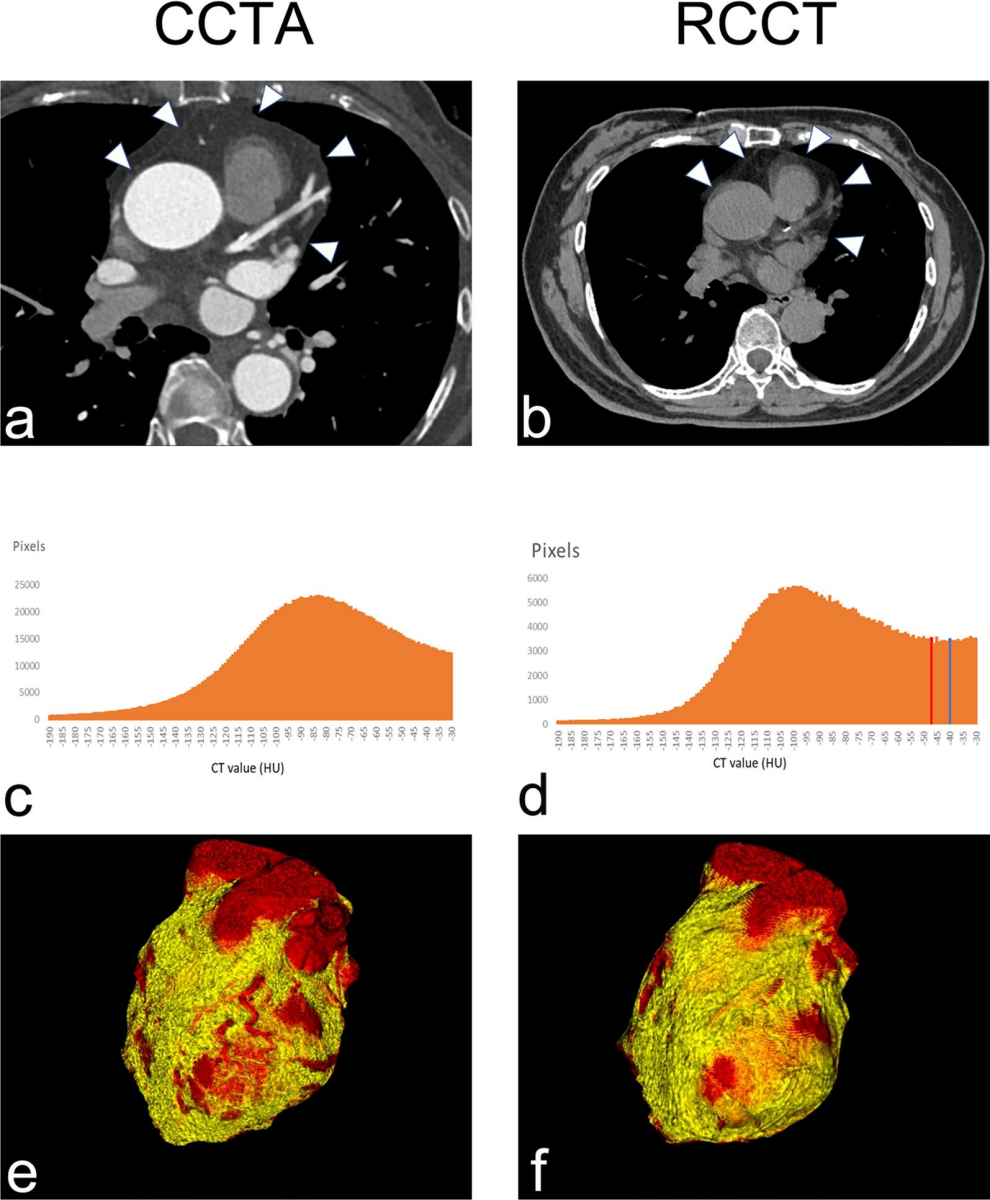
Visualization of the EAT in axial images, histograms and volume rendering. The pericardial structure (white arrowhead) in RCCT could be identified as clearly as that in CCTA (a, b). The similar pattern of CT value histograms extracted from pericardium segmentation from CCTA and RCCT both indicate that the adjustment of upper thresholds had a more obvious influence on the precision of fat volume measurements (c, d). The blue and red lines indicate the CT attenuation of − 40 HU (N40) and − 45 HU (N45), respectively. Volume rendering of the EAT and heart are displayed using the same threshold of (− 190 HU, − 30 HU) (e, f)
